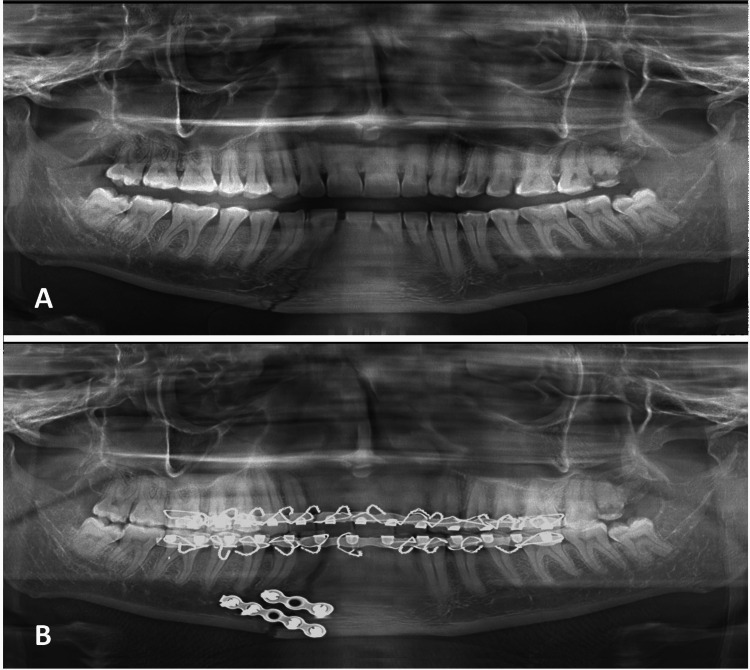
Orthopantomograph of mandibular body fracture treated by open reduction. (A) Pre-treatment. (B) Post-treatment.
